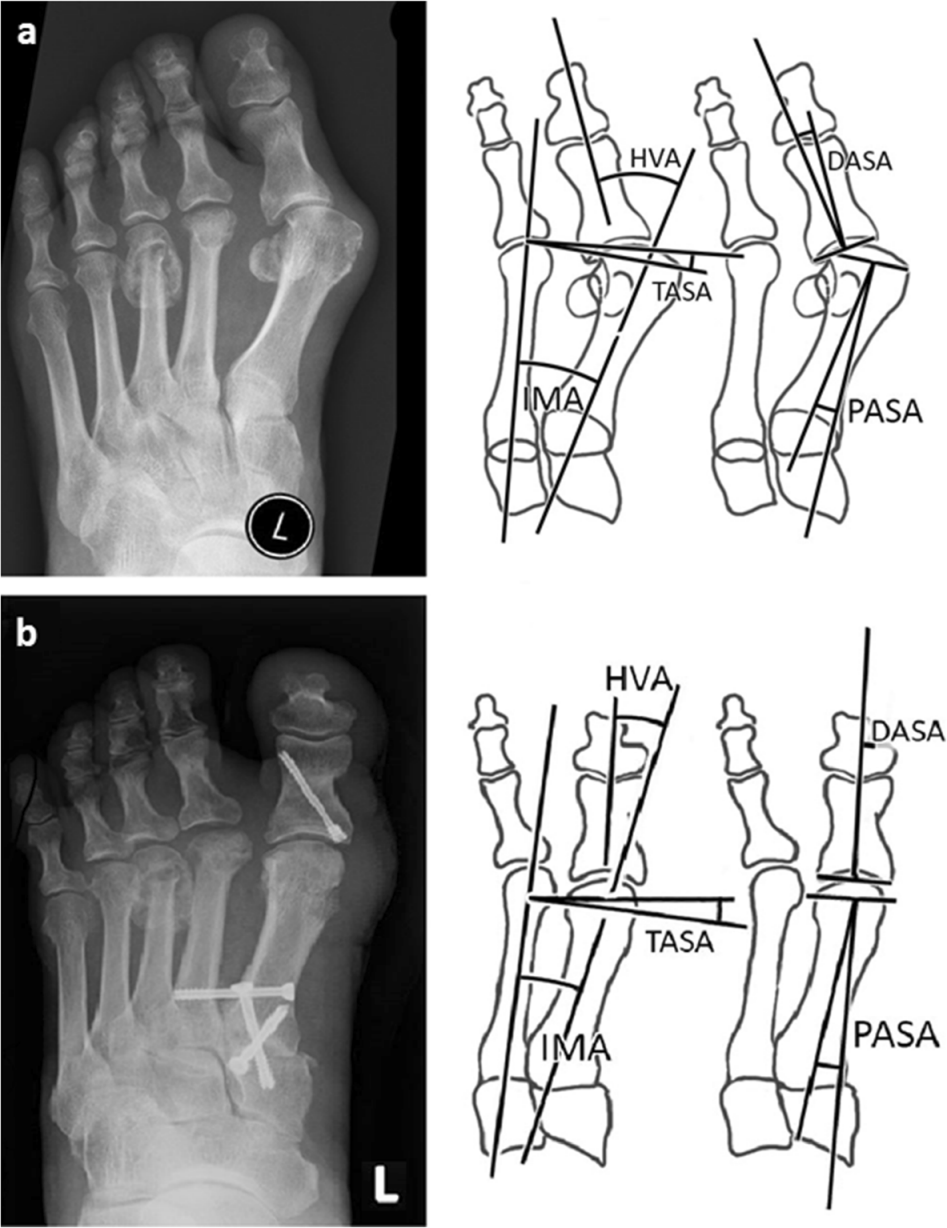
a, b Pre- and postoperative dorsoplantar radiographs of a patient who underwent Lapidus and Akin procedure. *Measurements of the HVA, TASA, DASA, PASA, and IMA. HVA – hallux valgus angle; TASA – tangential angle to the second axis; DASA – distal articular set angle; PASA – proximal articular set angle; IMA – intermetatarsal angle*
